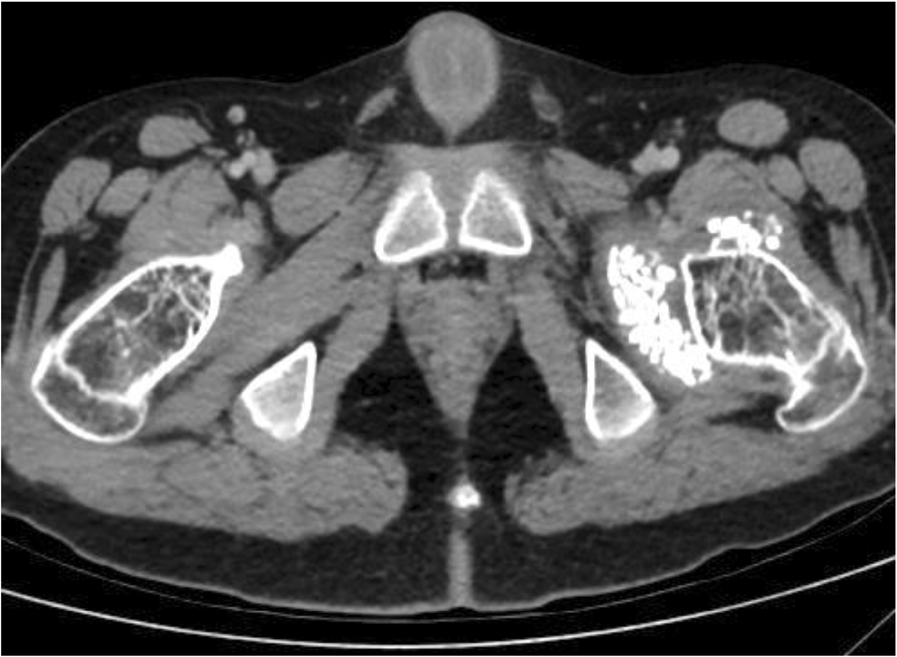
“Chondrocalcinosis” of the left hip joint capsule and generalized osteopenia on total body CT
